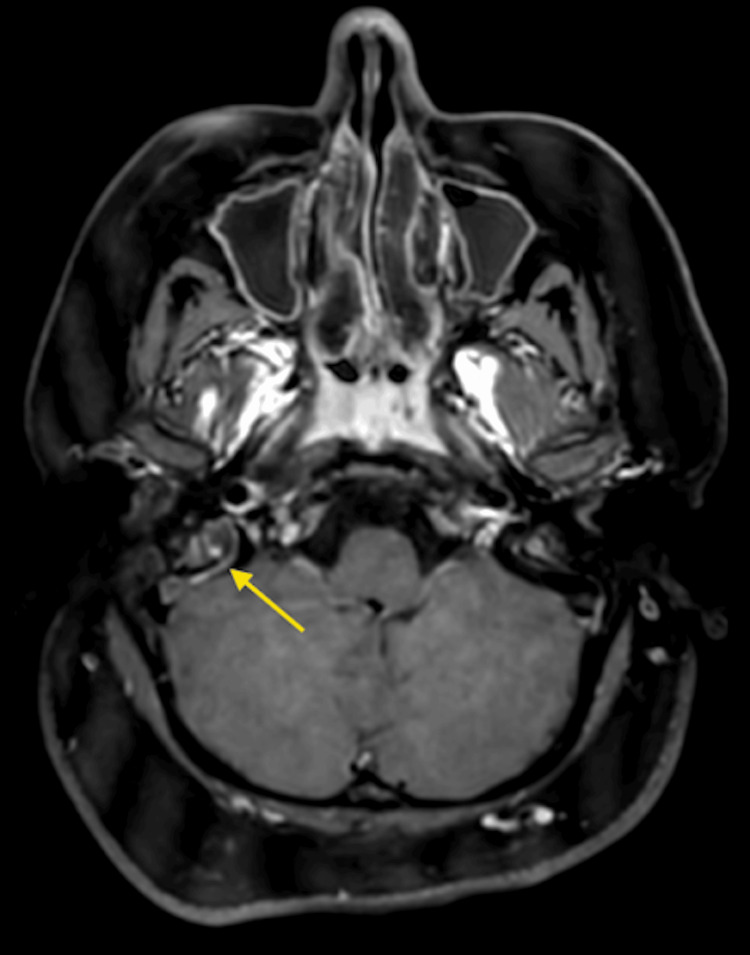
MRI Brain with gadolinium (T1, axial) shows abnormal hyperintense signal within the right distal transverse and sigmoid sinus (arrow) consistent with subacute thrombus.
